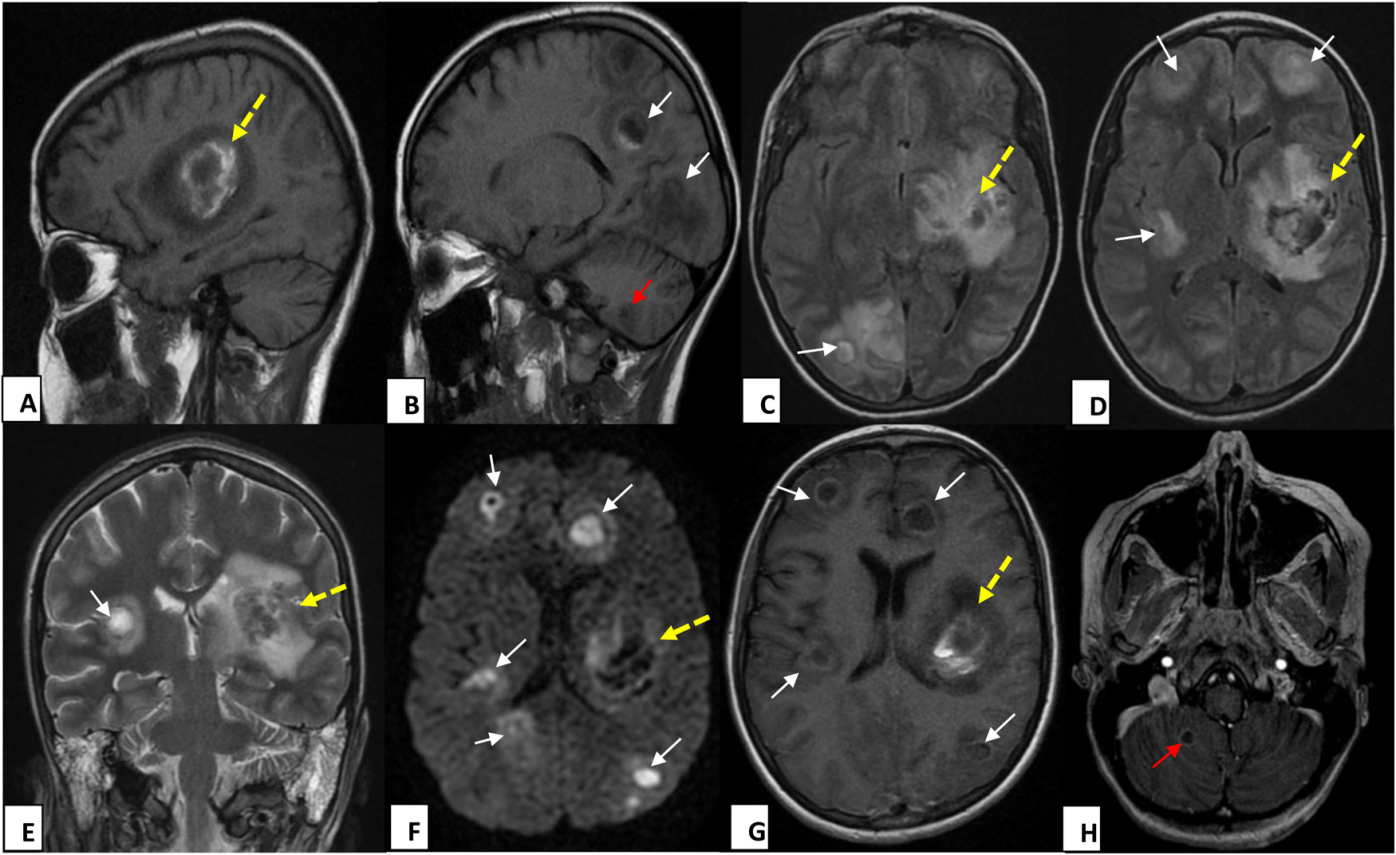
Brain MRI reveals a large, heterogeneous left capsulo-lenticular hematoma (yellow dashed arrows), characterized by peripheral hyperintensity on T1-weighted images (A, B) and significant perilesional edema on FLAIR (C, D) and T2-weighted sequences (E), causing a discrete mass effect. Concurrently, multiple cystic lesions that are hyperintense on T2/FLAIR, with hemorrhagic components on T1-weighted sequences and ring-like enhancement after contrast administration with ring-enhancing abscesses with irregular thick walls, are identified bilaterally at the gray–white matter junction (white arrows) and in the right cerebellar hemisphere (red arrows in B and H). These disseminated lesions exhibit restricted diffusion on DWI (F), consistent with septic emboli and purulent content.
